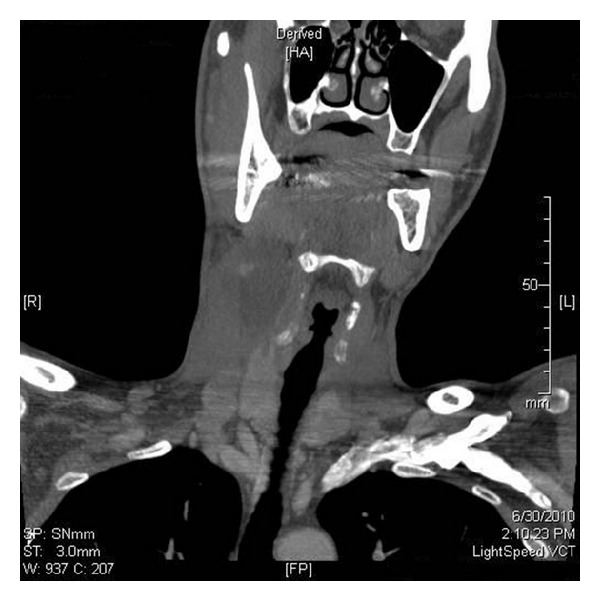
Coronal view of a CT scan with contrast showing right cystic neck mass. Lateral calcification corresponds to the area of malignancy.
